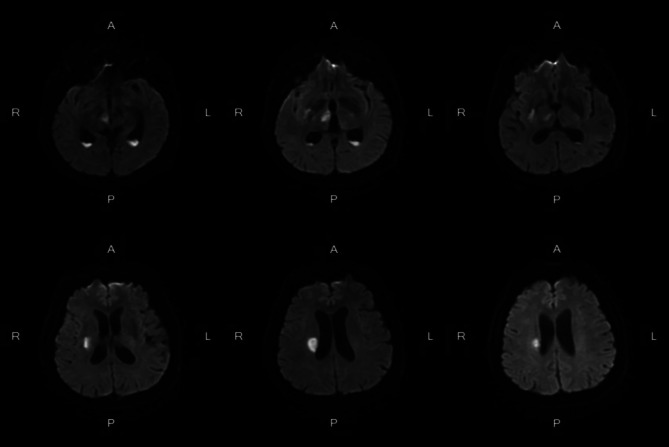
Brain cranial-enhanced magnetic resonance imaging
